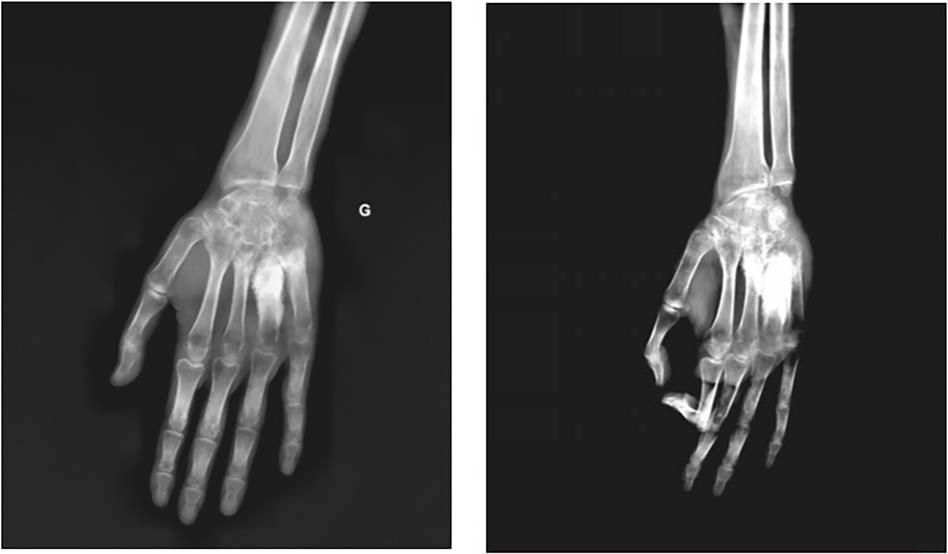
Radiographs of the left hand front (a) and oblique (b), showing cortical hyperdense lesion of the 3rd, 4th and 5th metacarpal bone, accentuated at the level of the 4th metacarpal with obliteration of the medulla, with a “dripping candle wax” appearance.
